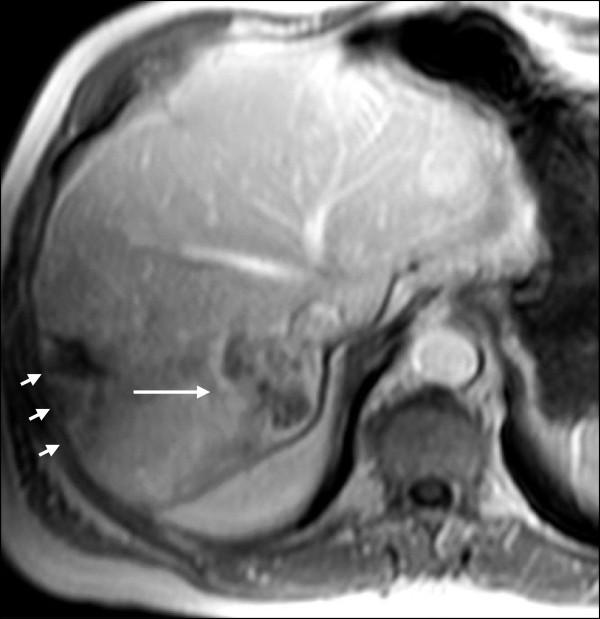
Magnetic resonance imaging of the transplant liver after selective internal radiation therapy (SIRT). Note the necrosis within the tumor center of the ICC as indicated by the long arrow. The small arrows point to fibrotic liver alterations due to SIRT.
